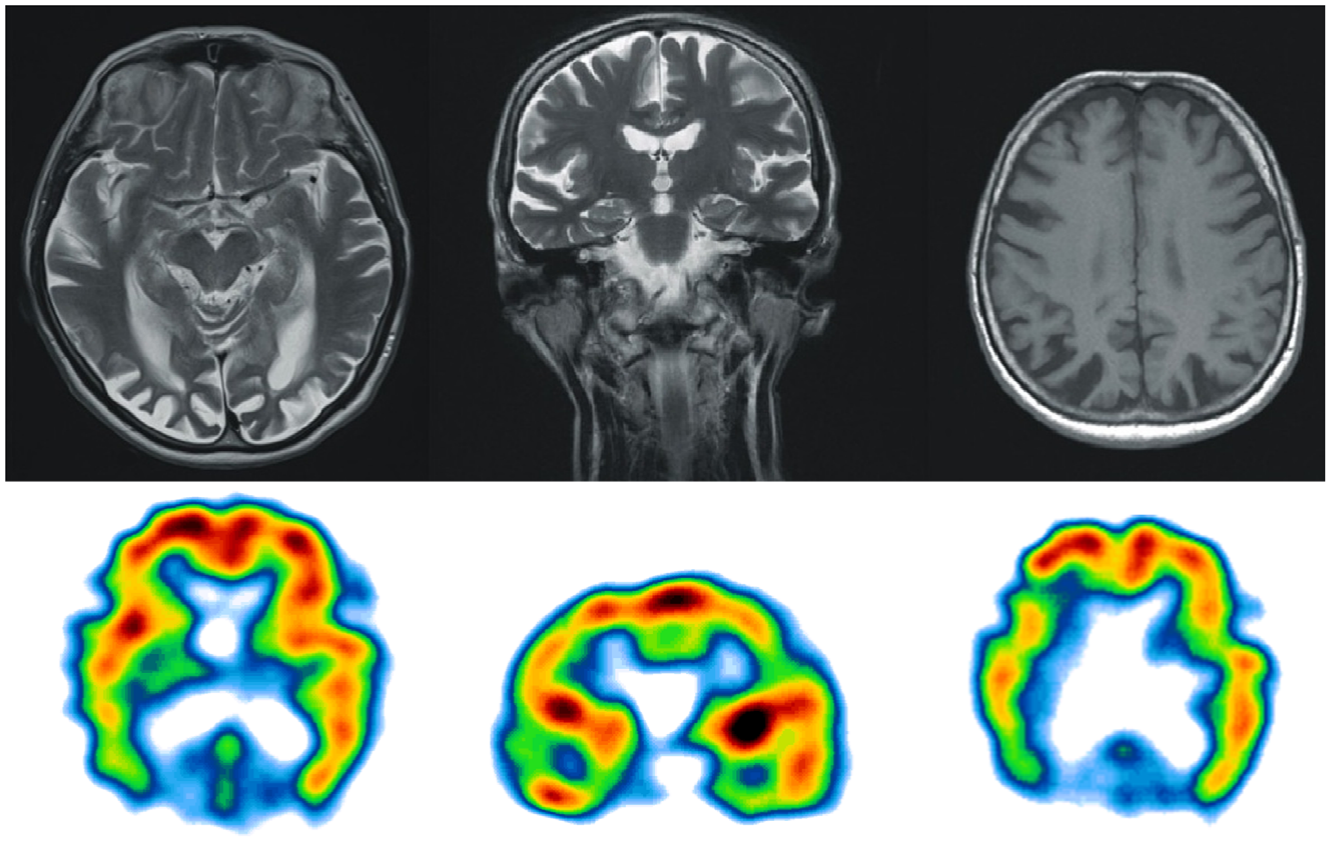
Results of MRI and SPECT examinations of the proband. Magnetic Resonance Imaging revealed marked cortical and subcortical atrophy within both occipital and parietal lobes bilaterally. The atrophy was less pronounced in the frontal and temporal lobes, and the hippocampal structures of the temporal lobes were mostly preserved. Single Photon Emission Computed Tomography demonstrated severe hypoperfusion within the parietal, occipital and temporal lobes bilaterally.
